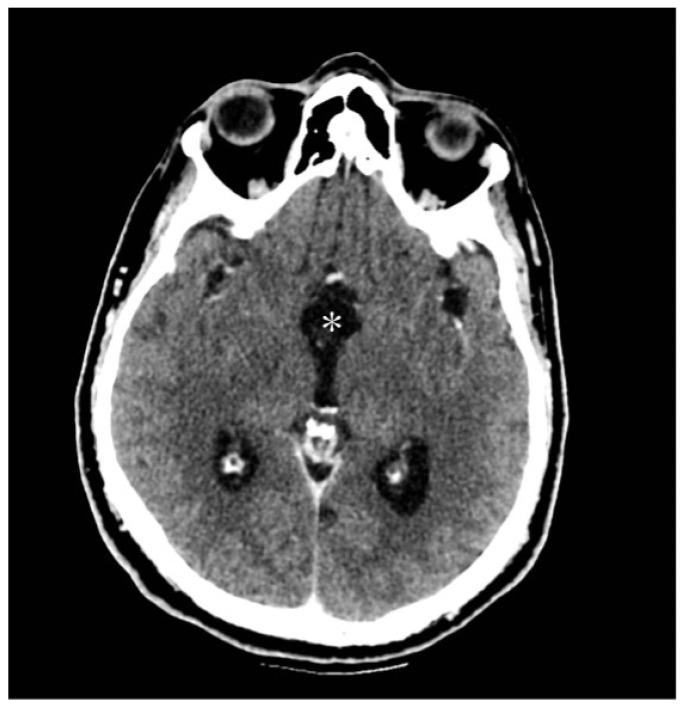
Postoperative CT brain scan showing a gross total resection (white asterisk) of the lesion with an initial decrease in ventricular size.
